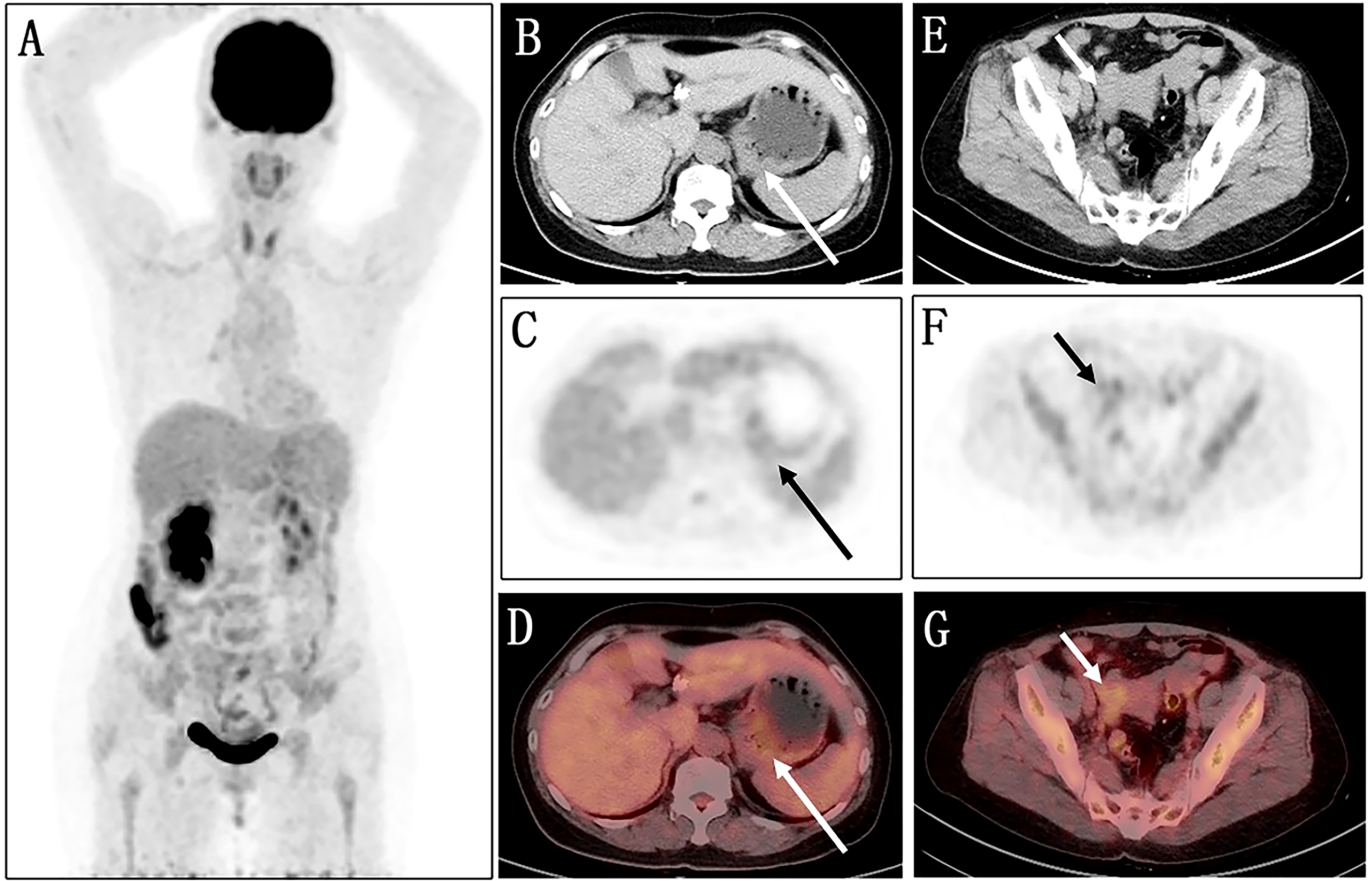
Images of ^18^F-FDG PET/CT. (A) No obvious abnormality is found in the maximum intensity projection (MIP) image. (B–D) The axial images of the abdominal cavity reveal slight FDG activity in the thickened gastric wall (long arrows; SUV_max_ 3.8). (E–G) Similarly, the axial images of the pelvic cavity show an abnormal mass with a slight FDG uptake in the right adnexal area (short arrows; SUV_max_ 3.0).
